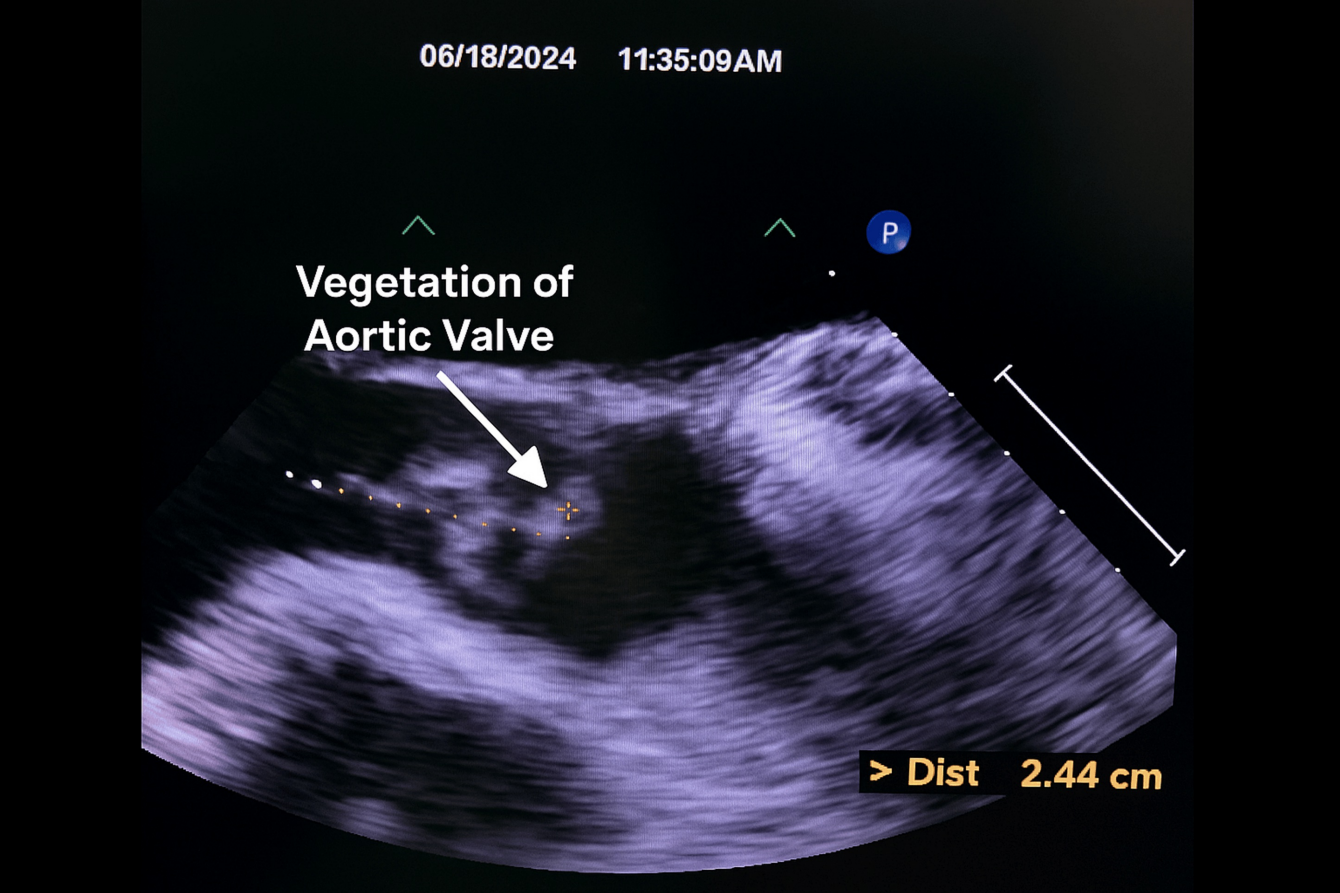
Right coronary cusp (RCC) aortic valve vegetation (transesophageal echocardiography)
